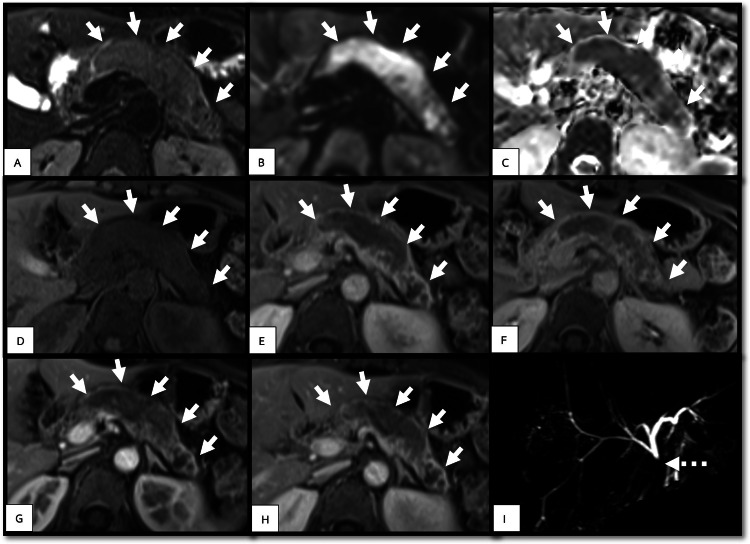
Abdominal MRI and MRCP (solid arrow, tumor; dotted arrow, common bile duct) The MRI (A, FS-T2WI; B, DWI; C, ADC; D, unenhanced FS-T1WI; E–H, dynamic contrast-enhanced FS-T1WI in sequential order; I, MRCP) revealed the mass to be isointense to slightly hypointense on FS-T1WI, slightly hyperintense on FS-T2WI, restricted diffusion on DWI, and hypointense on ADC. After contrast injection, the mass appeared hypovascular compared with the pancreatic parenchyma. The common bile duct in the area of the tumor was abruptly disrupted, according to the MRCP. (ADC, apparent diffusion coefficient; DWI, diffusion-weighted imaging; FS, fat suppression; MRCP, magnetic resonance cholangiopancreatography; MRI, magnetic resonance imaging; WI, weighted imaging).
